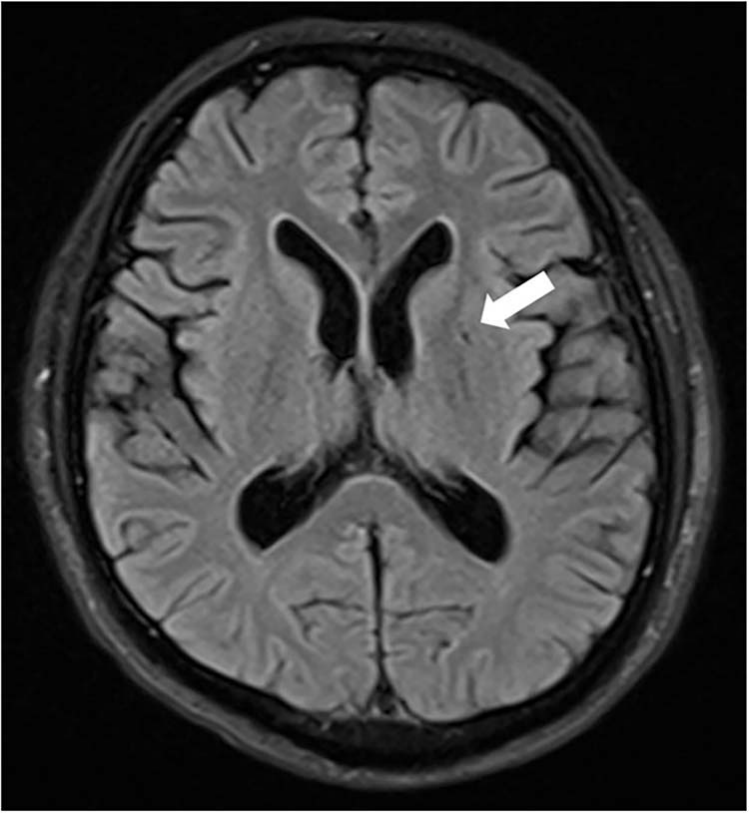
MR FLAIR image demonstrated one tiny hyperintensity lesion on basal ganglia (arrow) in patient’s first admission.
